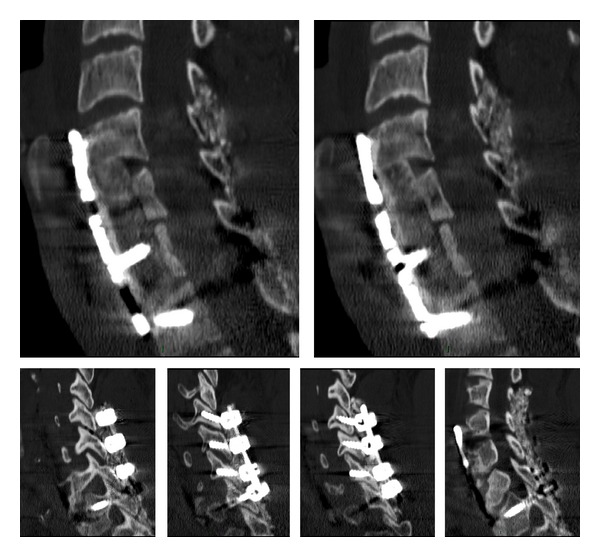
Postoperative CT scans showing posterior C4–C7 fixation and arthrodesis with lateral mass screwing, anterior C5-C6 corpectomy and tricortical bone grafting, and C4–C7 plating.
